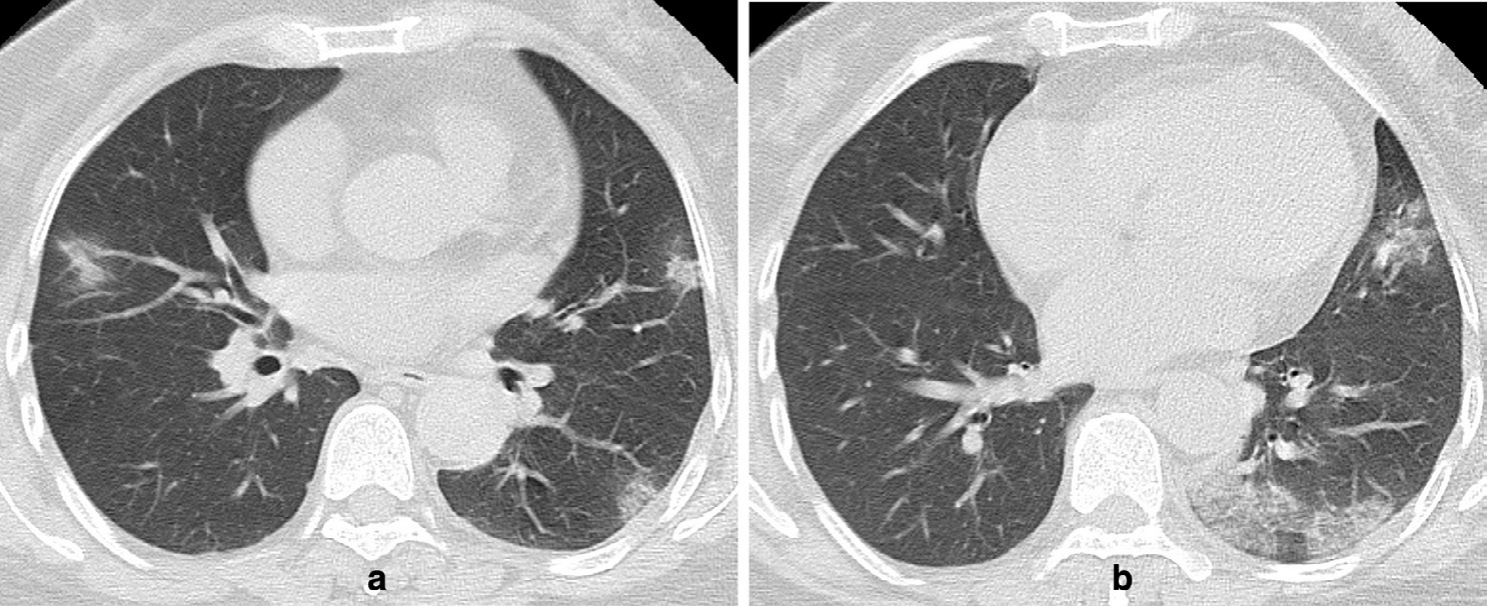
(a-b) A 56-year-old female with a history of travelling to Guangzhou from Wuhan presented with fever, cough and diarrhoea for 2 days. (a) (b) Axial thin-section non-contrast CT image showed bilateral peripheral/subpleural, patchy, pure/mixed GGOs with interlobular septal thickening and crazy-paving patterns. Two consecutive nasopharyngeal swabs for SARS-CoV-2 RNA(the interval between the two swabs more than 24 h) were negative.
